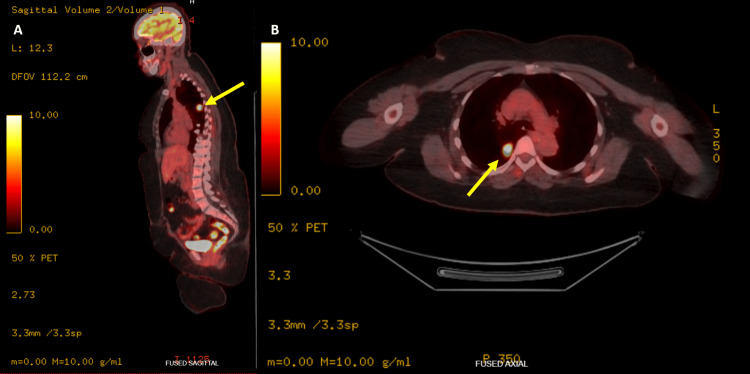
Positron emission tomography (PET) scan. F-18 FDG PET/CT scan (sagittal and axial views) showing intense FDG uptake tightly localized to the right lower lobe nodule (yellow arrows). The hypermetabolic activity strongly suggested malignancy, while the absence of regional nodal or distant metastasis confirmed very-limited-stage disease.
